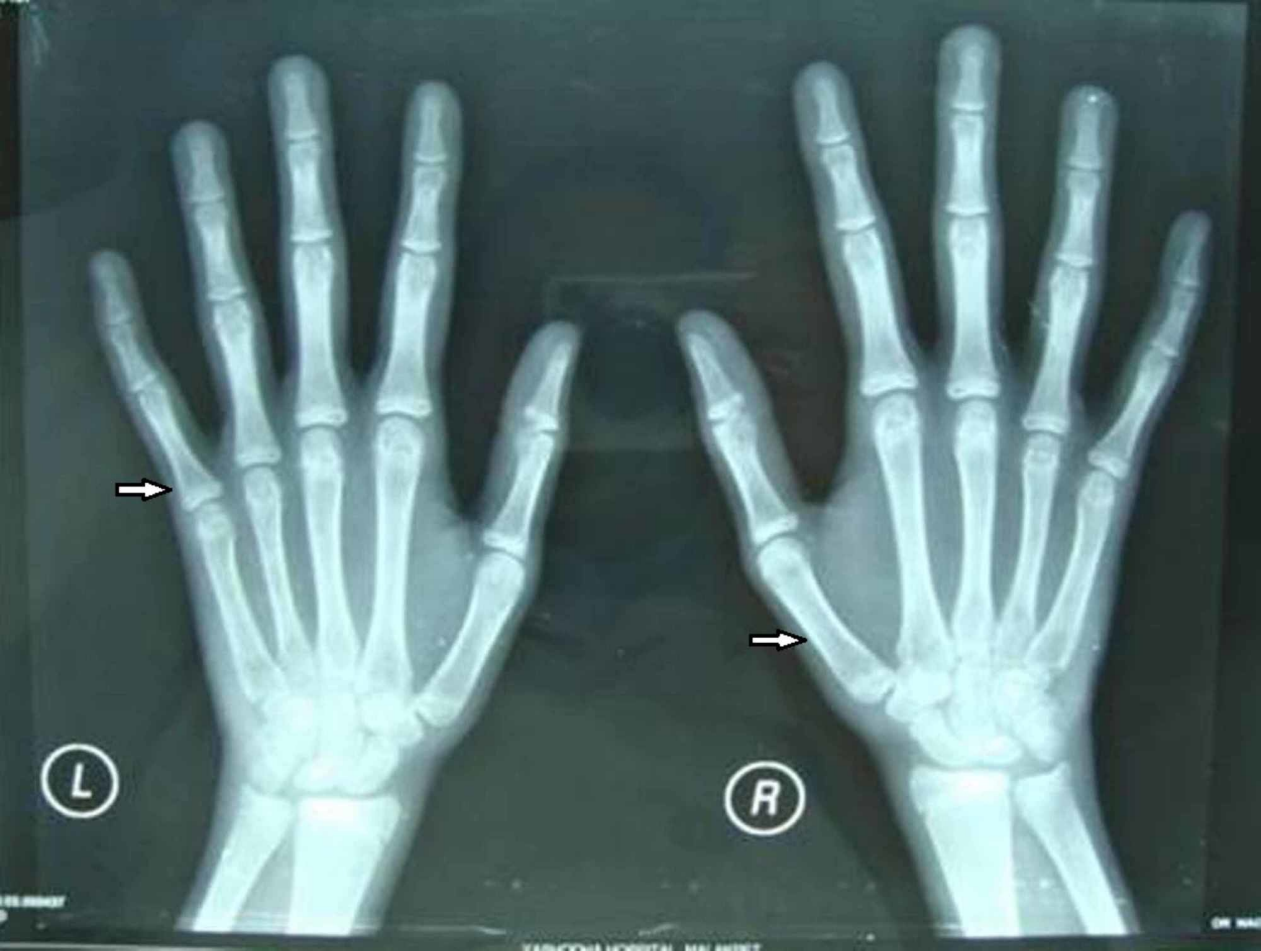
Hand-wrist X-ray of female patient
